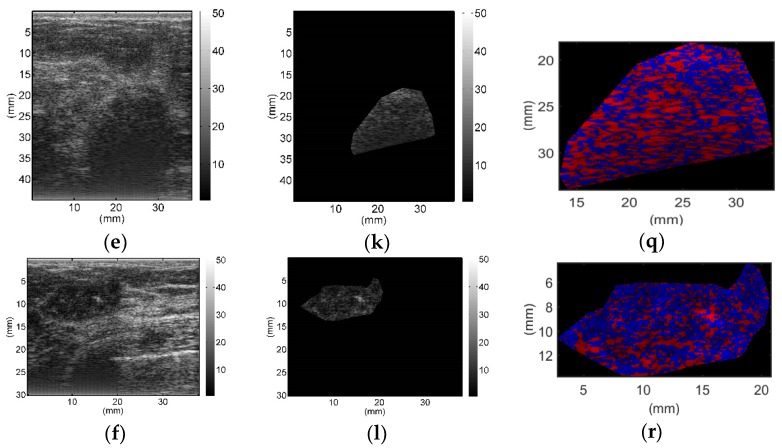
Typical B-mode (a–f), region of interest (g–l), and H-scan (m–r) ultrasound images of malignant breast tumors. H-scan images (m–r) correspond to the regions of interest of B-mode images (g–l).
